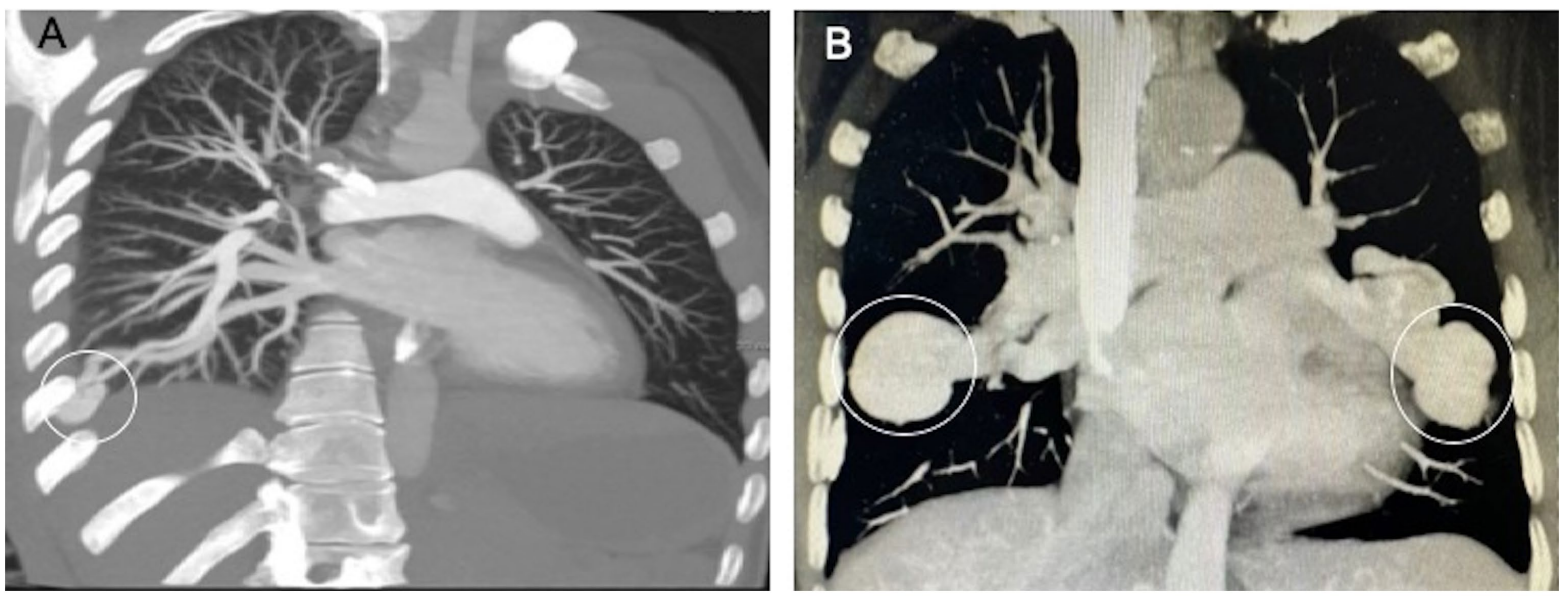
Typical CT appearance of a simple and a complex PAVM. (A) The simple PAVM. (B) The complex PAVMs. (White circle, aneurysmal sac).
